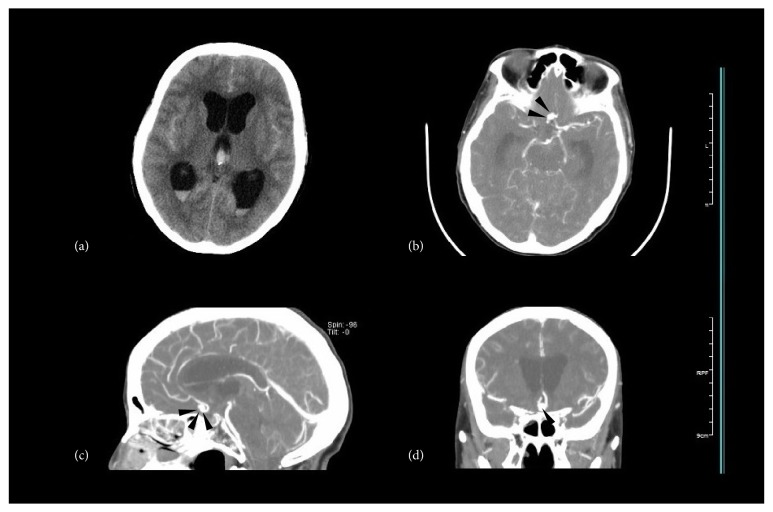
This picture shows a case of acute HCP induced by aneurysmal SAH, typically with an IVH. It happened as soon as the occurrence of SAH. (a) The CT scans above show widely hemorrhagic sulci and arachnoid cisterns with dilated lateral and third ventricles containing blood. (b), (c), and (d) Immediate CTA after admission locates the culprit aneurysm on the anterior communicating artery (marked by black arrows).
